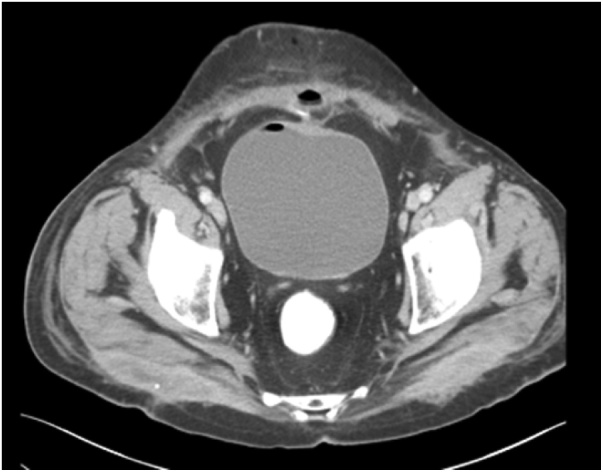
Abdominal CT with IV and oral contrast showed air pocket in bladder and ileo-cutaneous fistula.
